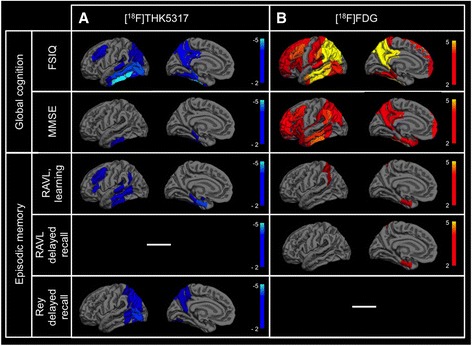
Cortical regions of interest where PET tracer retention was significantly associated with cognition in AD patients, after adjusting for delay (not corrected for multiple comparisons). Linear models assessed the association between cognitive performance using five different tests (each *row*) and (a). [^18^F]THK5317 retention (DVR) or (b). [^18^F]FDG uptake (SUVR). Regions are defined according to the Harvard-Oxford atlas. T-values for significant negative associations are displayed with a *blue color scale* and T-values for significant positive associations are displayed with a *red color scale. FSIQ* full-scale intelligence quotient, *MMSE* mini mental state examination, *RAVL* Rey auditory verbal learning test, *Rey* Rey-Osterrieth complex figure test
